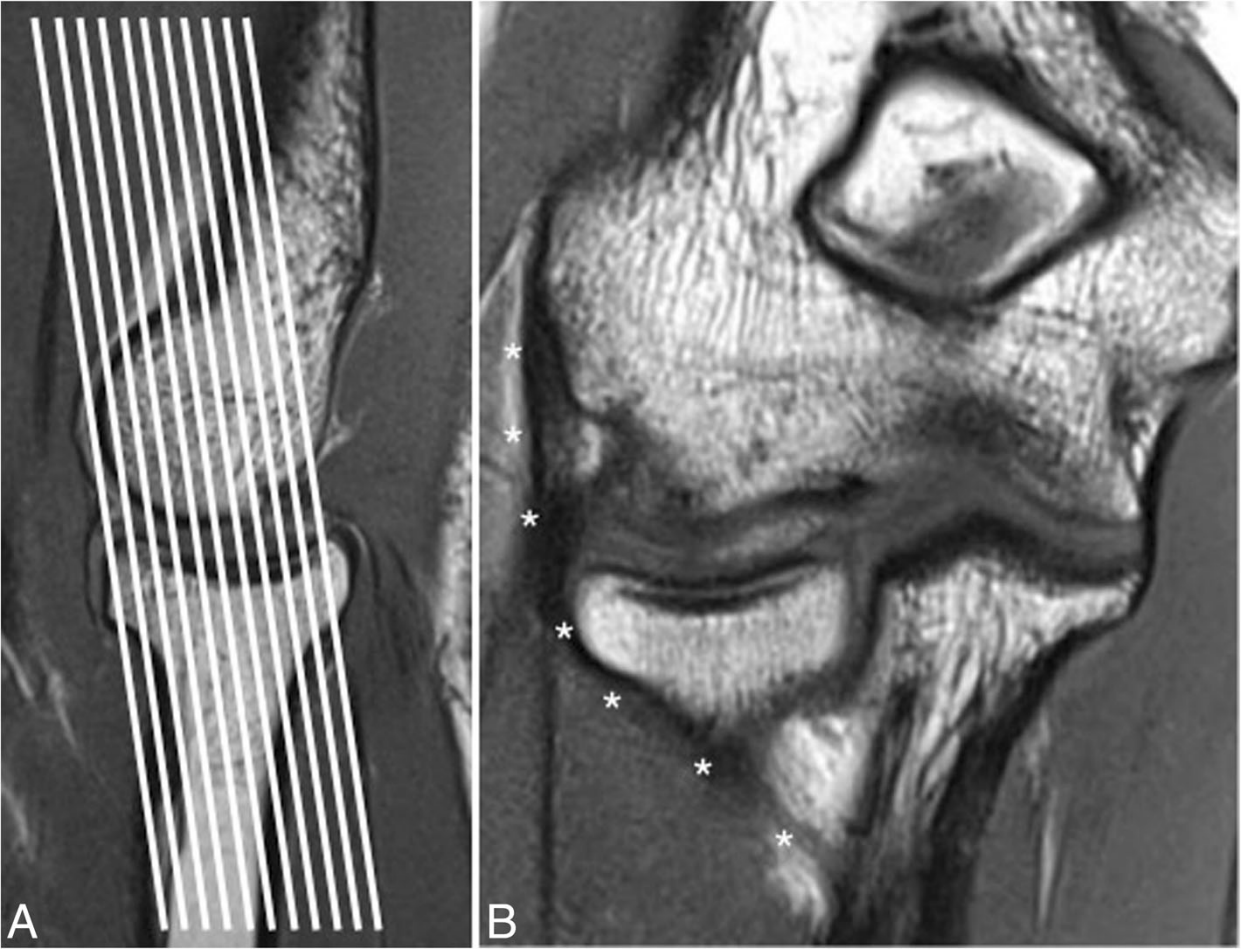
Modified oblique coronal plane of the elbow used to optimally visualize the collateral ligaments. a Sagittal T1-weighted MRI showing the modified oblique coronal plane (multiple white lines) oriented 20–30° posterior to the long axis of the humeral diaphysis. b Coronal T1-weighted MRI showing the intact lateral ulnar collateral ligament (white asterisks)
